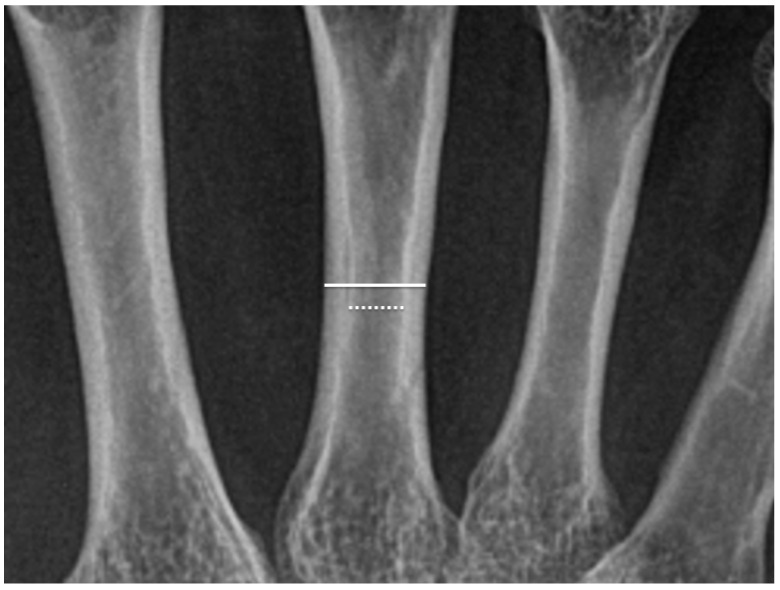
In this radiographic image of the metacarpal bone, the solid white line (upper line in the figure) represents the periosteal (outer) diameter, and the dotted white line (lower line in the figure) represents the endosteal (inner) diameter. These measurements are taken at the midpoint of the diaphysis. Cortical thickness is calculated as the difference between the outer and inner diameters, and the cortical thickness rate (CTR) is calculated by dividing the cortical thickness by the outer diameter.
